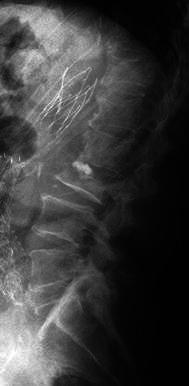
Lumbar spine X-ray showing vertebroplasty procedure performed 3 months after aneurysm repair
